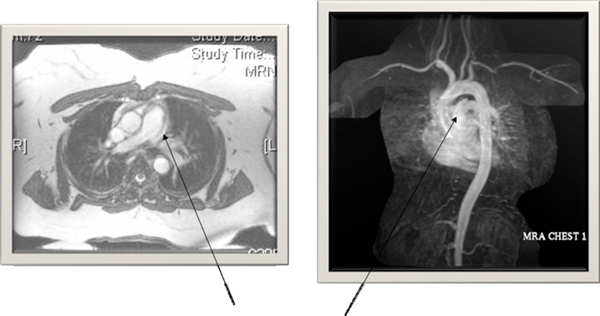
Cardiac magnetic resonance imaging demonstrating an intimal dissection flap of the main pulmonary artery.
